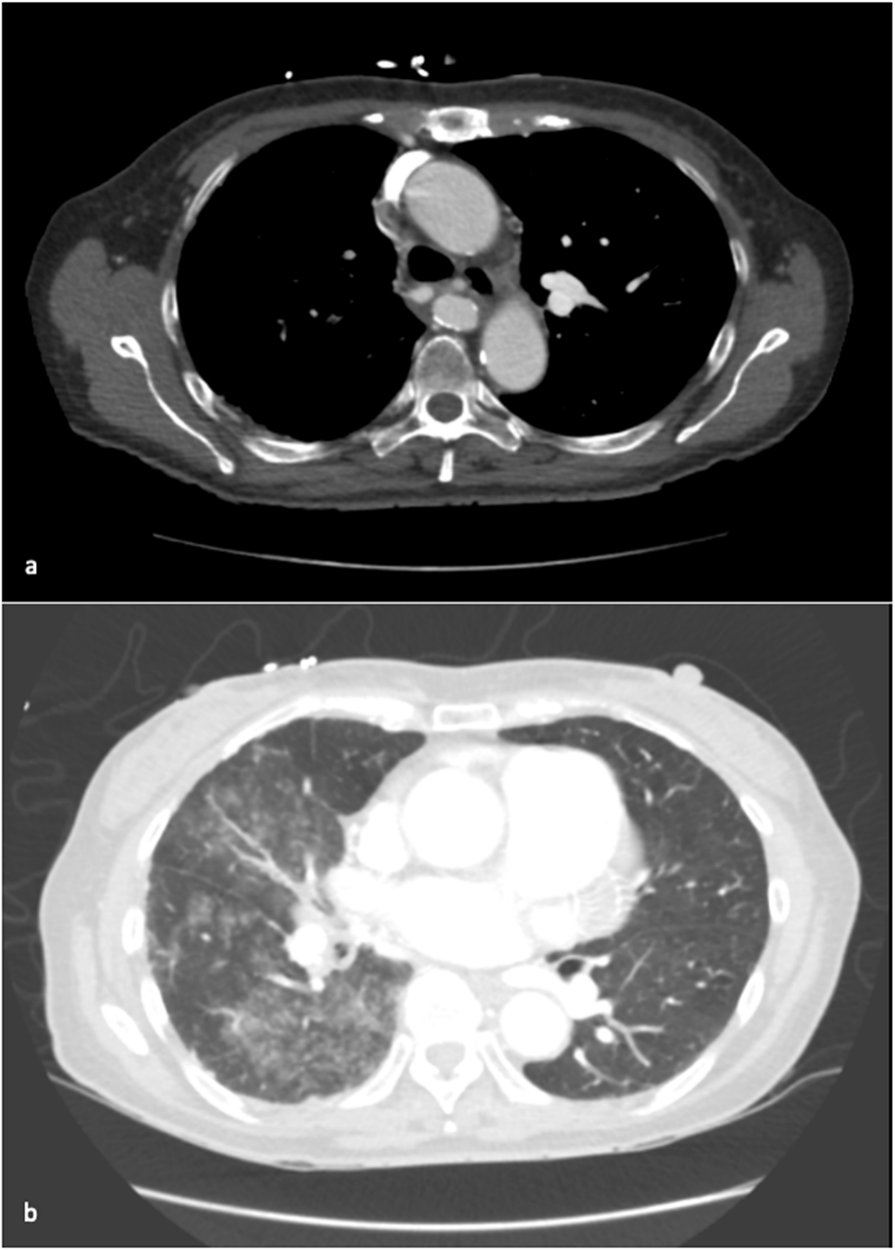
a CT bronchial-angiogram demonstrates aneurysm of the ICBT and hypertrophy of bronchial collaterals at the hilum; b CT bronchial-angiogram lung window demonstrates ground glass attenuation throughout right mid/lower lobes, consist with pulmonary haemorrhage at the time of presentation
